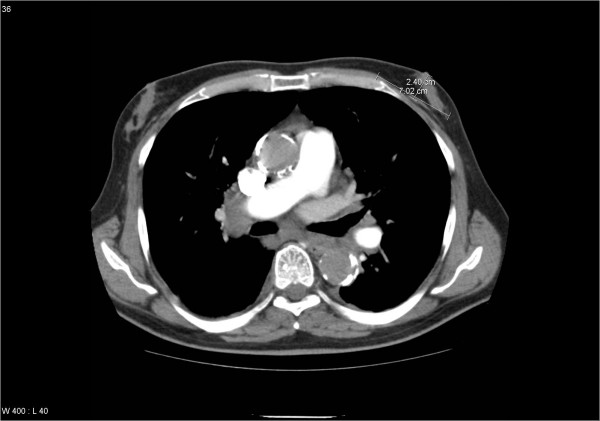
Axial contrast-enhanced computed tomography scan at the level of the left mamilla displaying both the distance between the skin surface and the pectoral musculature (2.4 cm) and the field size of 7 cm. Note that only very low heart exposure results from electron beam irradiation at this level, i.e. the center of the field.
